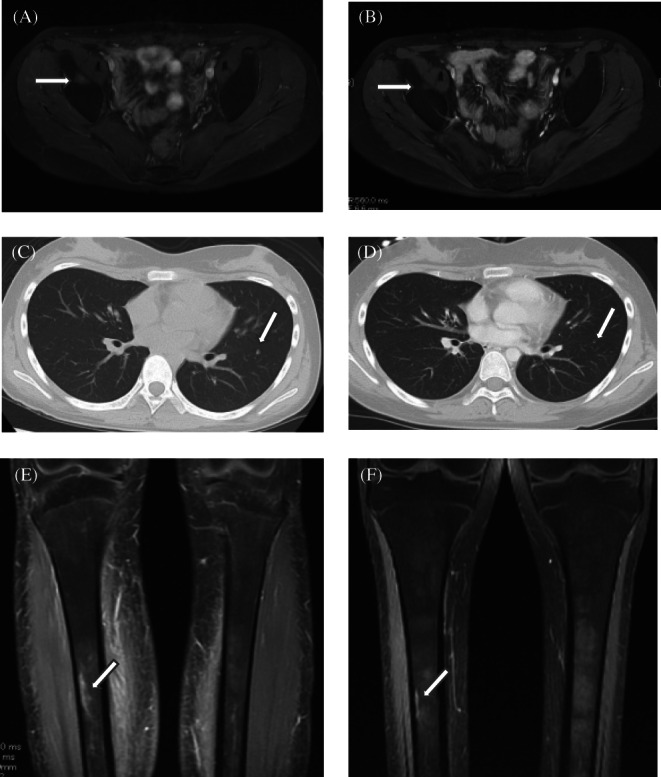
(A) Patient 3. Magnetic resonance imaging of the pelvic bone by short TI inversion recovery (STIR) prior to TCR‐haplo‐SCT. Arrow shows the bone metastasis of the right ilium. (B) Patient 3. Magnetic resonance imaging of the pelvic bone by STIR at 3 months after TCR‐haplo‐SCT. The size of the bone metastatic lesion in the right iliac bone was markedly reduced (arrow). (C) Patient 5. Computed tomography slice of lung prior to TCR‐haplo‐SCT. Arrow shows the lung metastasis of the left lung. (D) Patient 5. Computed tomography slice of lung at 3 months after TCR‐haplo‐SCT. The size of the left lung metastasis was reduced (arrow). (E) Patient 6. Magnetic resonance imaging of tibia by STIR prior to TCR‐haplo‐SCT. Arrow shows the bone metastasis of the right tibia. (F) Patient 6. Magnetic resonance imaging of tibia by STIR at 3 months after TCR‐haplo‐SCT. The lesion of the right tibia metastasis was reduced (arrow)
